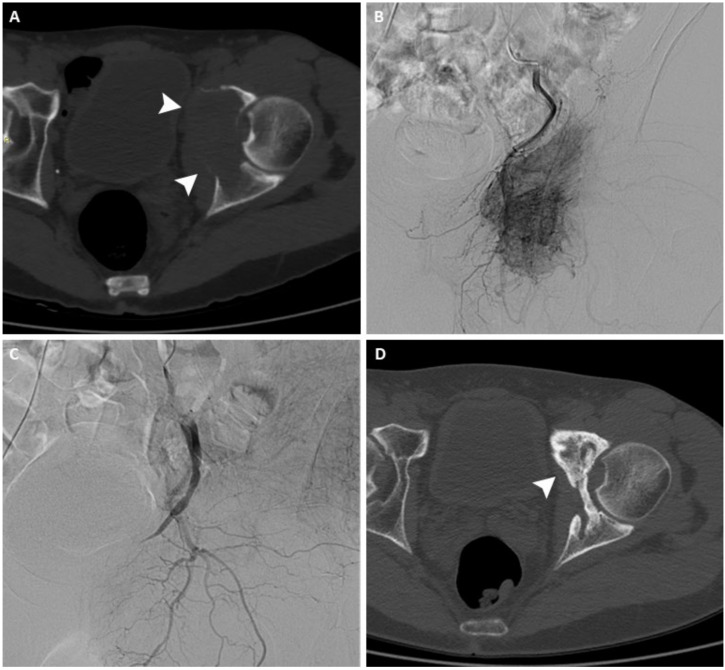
(A) Axial CT scan of the pelvis of a 63-year-old man with a painful left acetabular bone metastasis from kidney cancer (arrowheads). (B) Arteriography shows pathological vascularization originating from branches of the internal iliac artery. (C) After arterial embolization, arteriography demonstrates complete occlusion of the feeding vessels. (D) Axial CT scan performed 12 months after treatment shows signs of re-ossification and local disease control (arrowhead).
